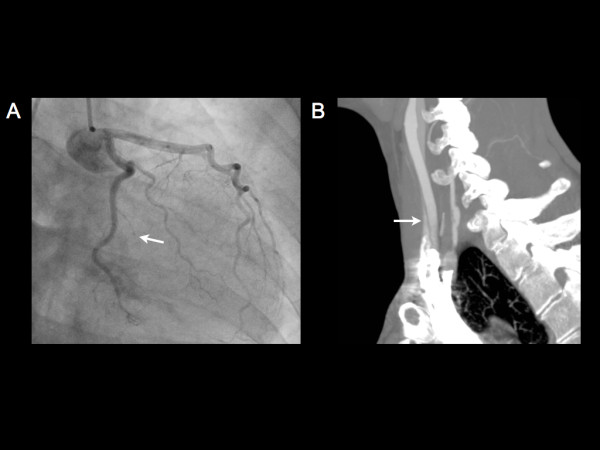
A) Diffuse tapering and occlusion in the mid body of the obtuse marginal 2 consistent with a dissection and occlusive intramural hematoma; B) Sagittal oblique maximum intensity projection reformation of a CT angiogram of the carotid and vertebral arteries demonstrates a focal dissection with associated localized aneurysmal dilatation of the V1 segment of the right vertebral artery.
